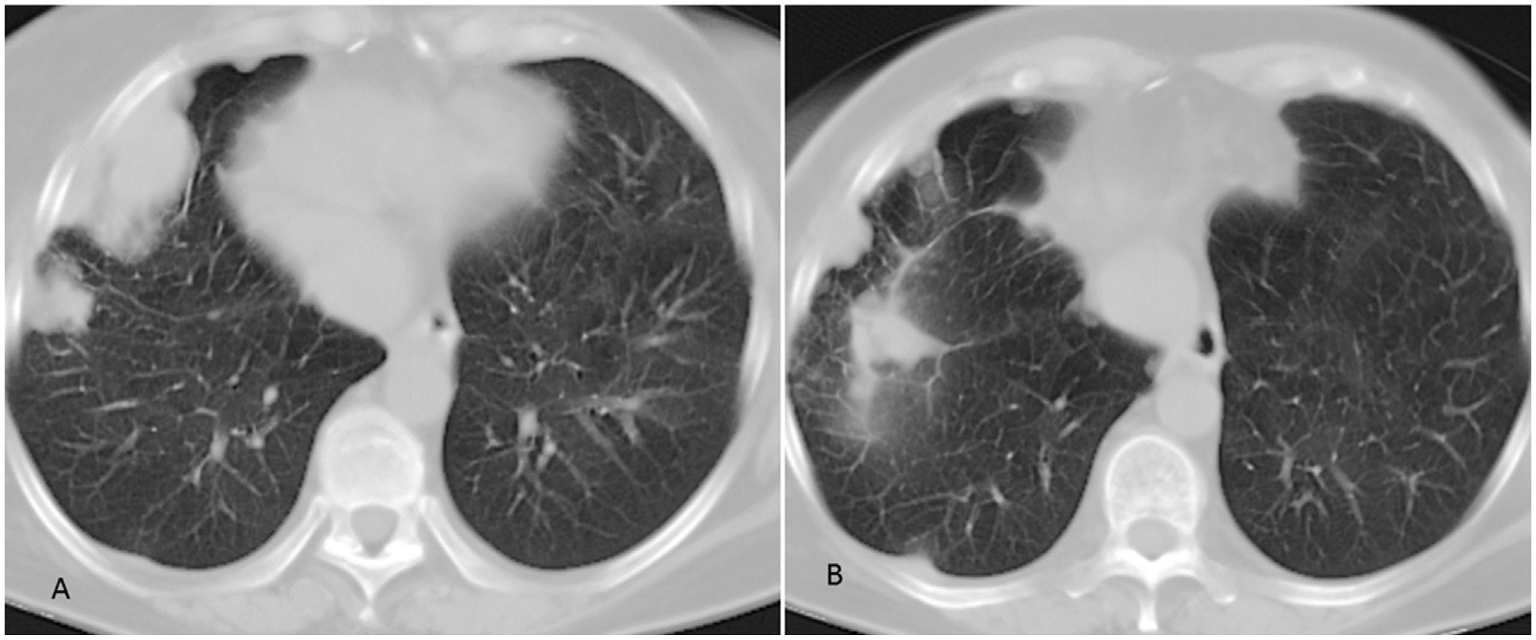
Chest CT scan of the patient. (A) Pulmonary lesions of the right lung. (B) Partial response of pulmonary lesions following 6 months of treatment with pazopanib.
